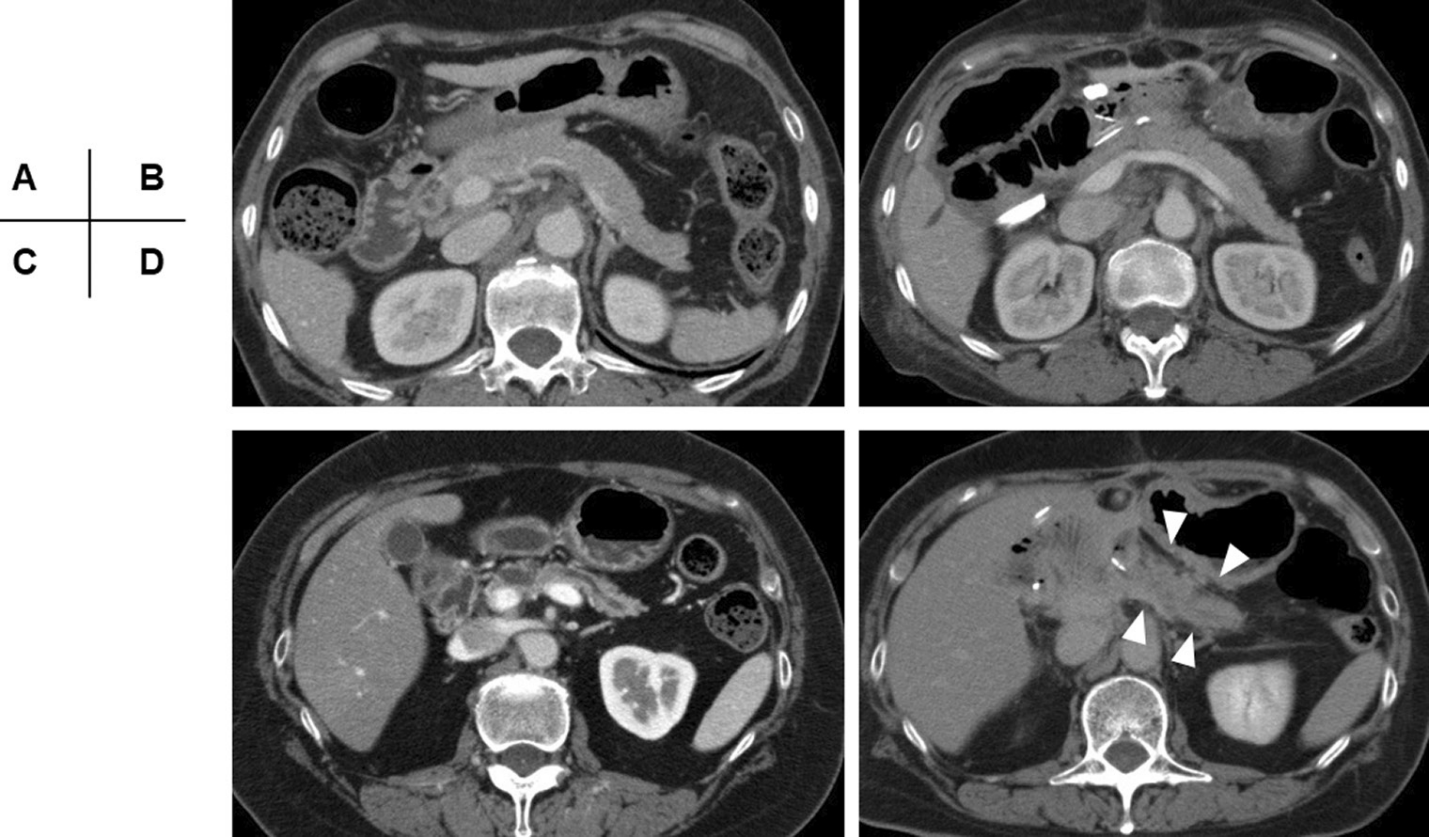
Preoperative (A,C) and postoperative (B, D) CT findings in patients who underwent PD. Preoperative (A) and postoperative (B) CT findings in a patient who did not develop acute pancreatitis after PD. (B) The remnant pancreas on the CT examination taken on POD 8 has not changed compared with the pancreas on preoperative CT. Preoperative (C) and postoperative (D) CT findings in the patients who developed mild acute pancreatitis after PD. the remnant pancreas on the CT taken on POD 24 demonstrates interstitial edema and inflammatory changes in peripancreatic fat (arrowheads). CT, computed tomography; PD, pancreaticoduodenectomy; POD, postoperative day
